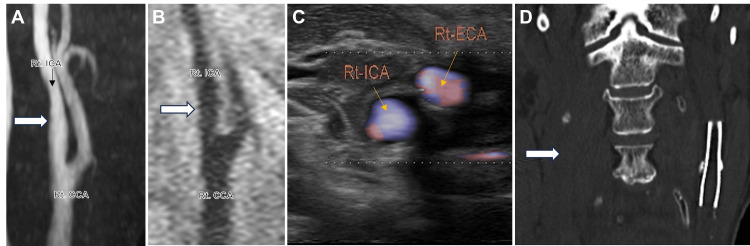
Radiological images after the second intervention (A) Cervical MRA reveals the resolution of the previously noted stenosis in the right ICA (arrow). (B) Black-blood T1-weighted image confirms the disappearance of the previously noted mixed-intensity signal (arrow). (C) Carotid duplex ultrasonography shows the resolution of luminal stenosis of the right ICA with a normalized peak systolic velocity of 60 cm/second. (D) Coronal non-contrast cervical CT reveals the absence of the previously visualized calcified plaque at the right carotid bifurcation (arrow). CCA: common carotid artery, ECA: external carotid artery, ICA: internal carotid artery; MRA: magnetic resonance angiography; CT: computed tomography
